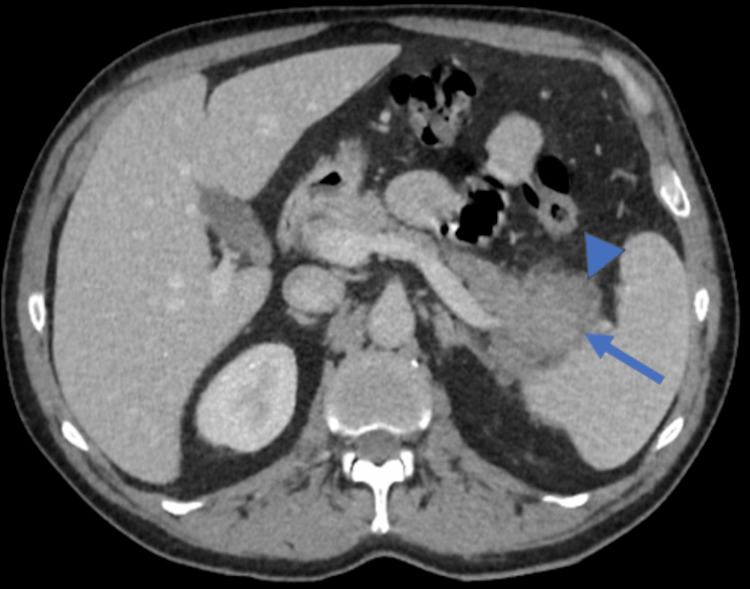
Initial contrast-enhanced computed tomography (CECT) Soft mass around the pancreatic tail (arrowhead). We can notice the moderate enlarged pancreatic tail within the mass, compared to its body (arrow).
